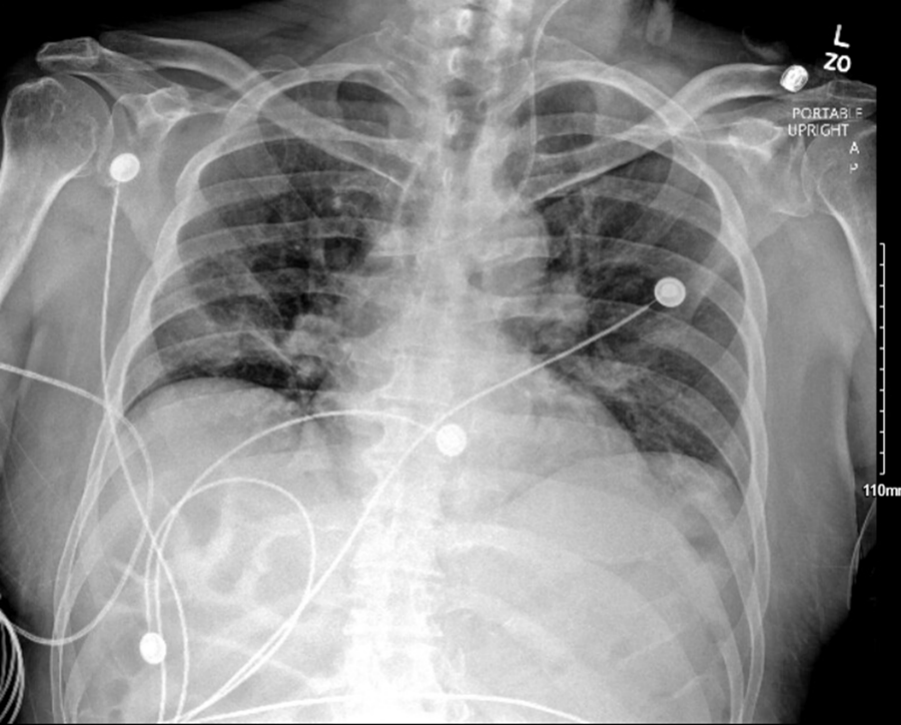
Chest Xray showing the central venous catheter crossing the midline to the right hemithorax and pointing toward the right upper lung.
